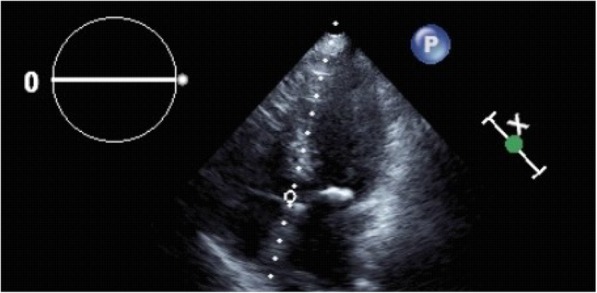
Transthoracic echocardiogram revealing apical hypokinesis
